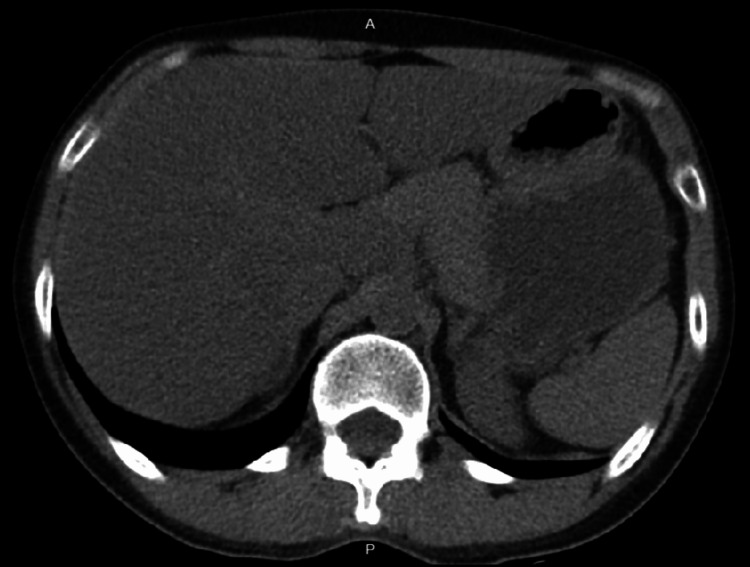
CT abdomen shows fatty infiltration of the liver without CBD dilatation CT: computed tomography; CBD: common bile duct.
